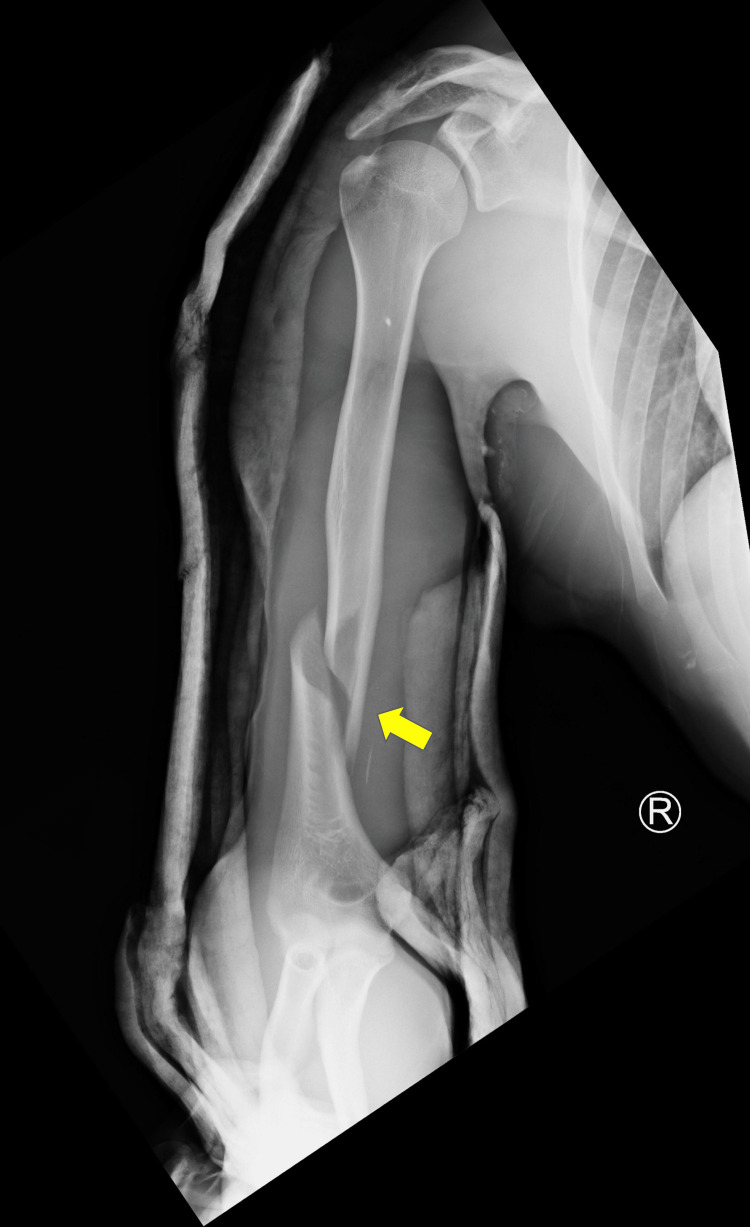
An X-ray on admission showing a distal, one-third spiral humeral fracture (arrow)
